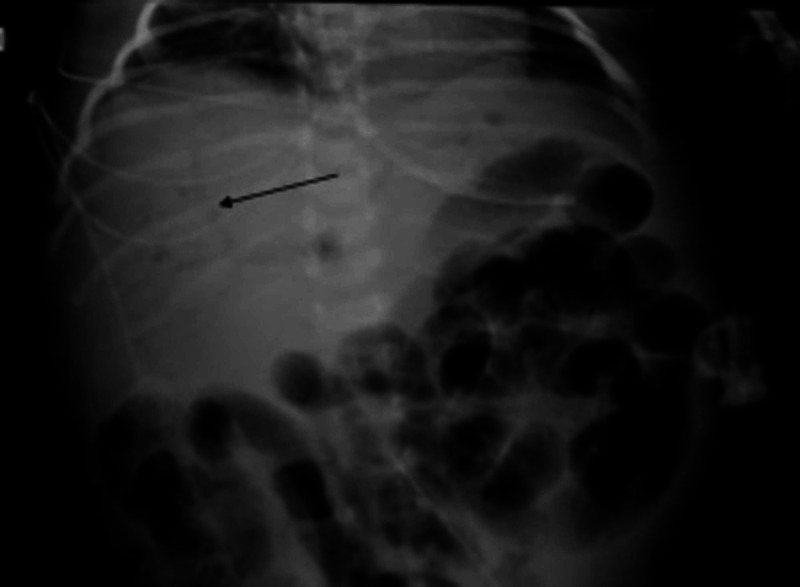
Abdominal X-ray showing portal venous gas (arrow).
